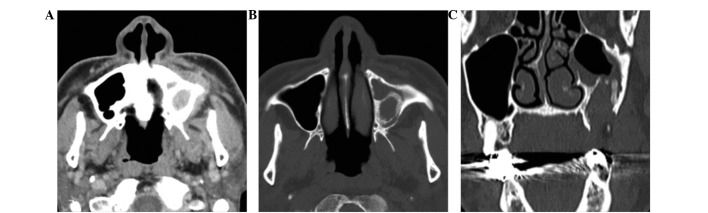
Computed tomography images. (A) Axial soft tissue window images revealed a cystic lesion extending from the left maxillary alveolar area to the maxillary sinus. (B and C) Axial and coronal bone window images revealed the thickened floor of the antrum, which was elevated. (C) A section of the elevated sinus floor was destroyed.
